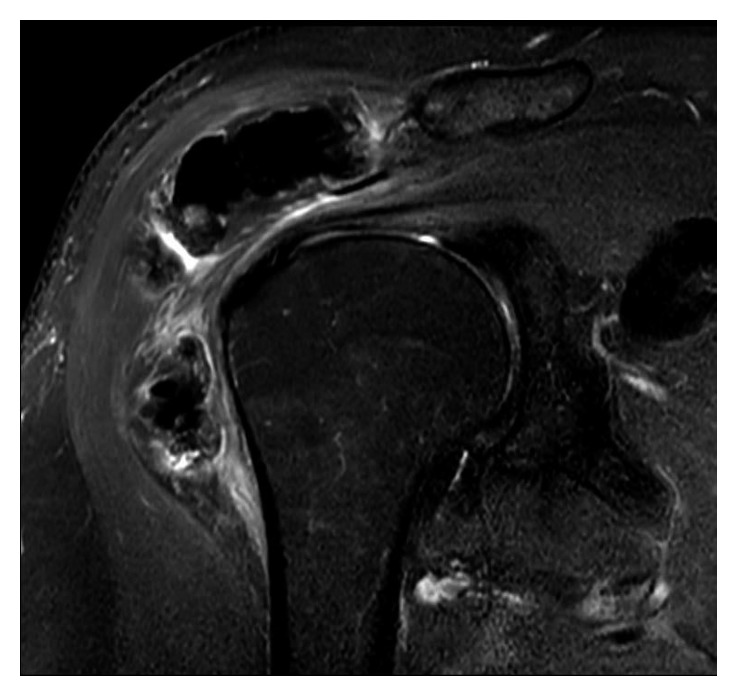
Magnetic resonance imaging of the left shoulder. Coronal T2 fat suppression image showing low-signal intensity calcific foci within and beneath the deltoid muscle with no intra-articular involvement.
